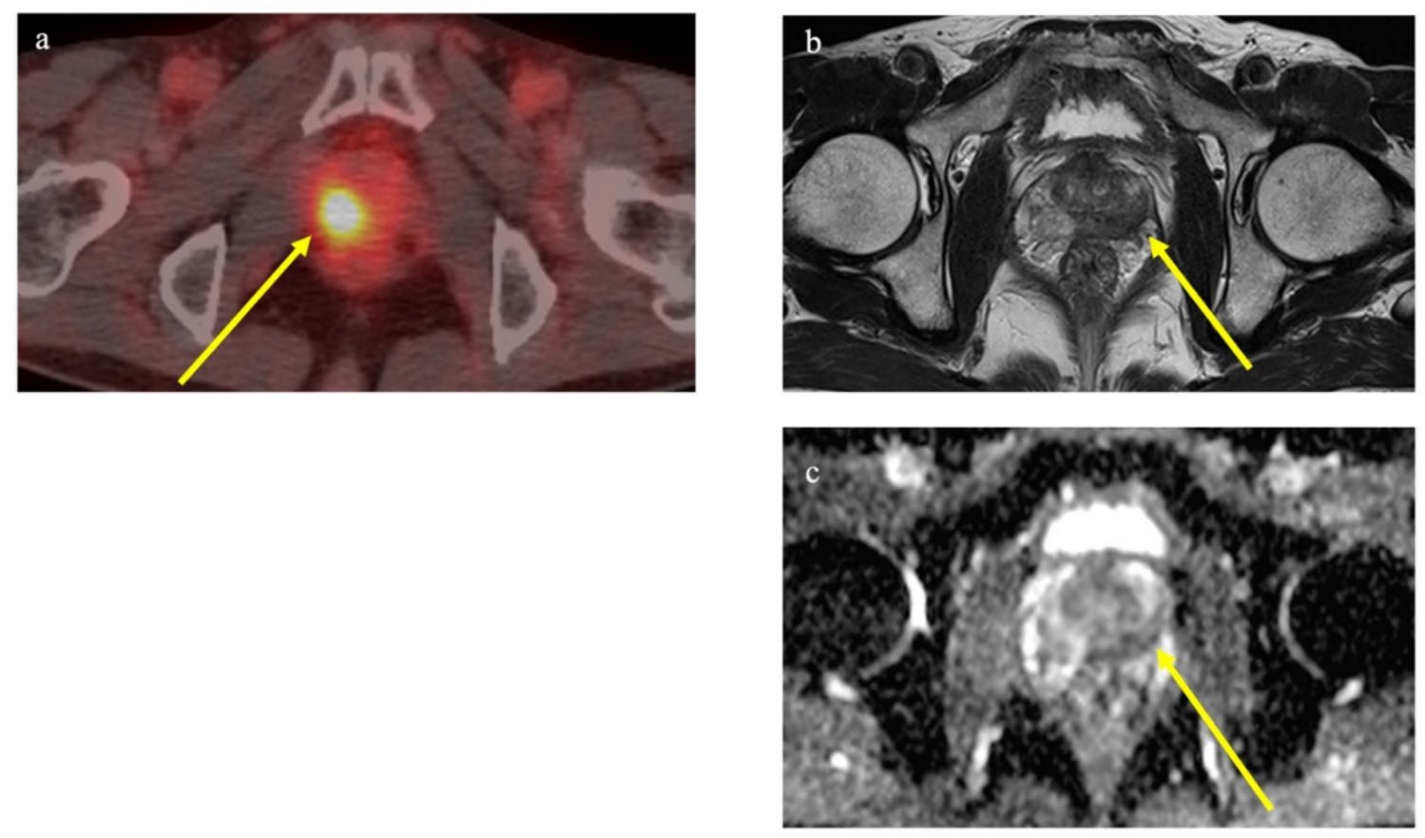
PET/CT using ^18^F-FSU-880 in a 79-year-old man with biochemical recurrence after radiation therapy (cT3bN0, Gleason score 4 + 4). PSA level increased to 4.4 ng/ml. Focal uptake was observed in the right side of the prostate, suggesting local recurrence (a). The initial MRI (conducted 12 years earlier) showed a signal-hypointense tumor on both T2-weighted imaging and ADC mapping in the peripheral zone of the left prostate, distinct from the recurrence site (b, c). a Transaxial PET/CT fusion image. b Transaxial T2-weighted MR image. c Transaxial ADC mapping
